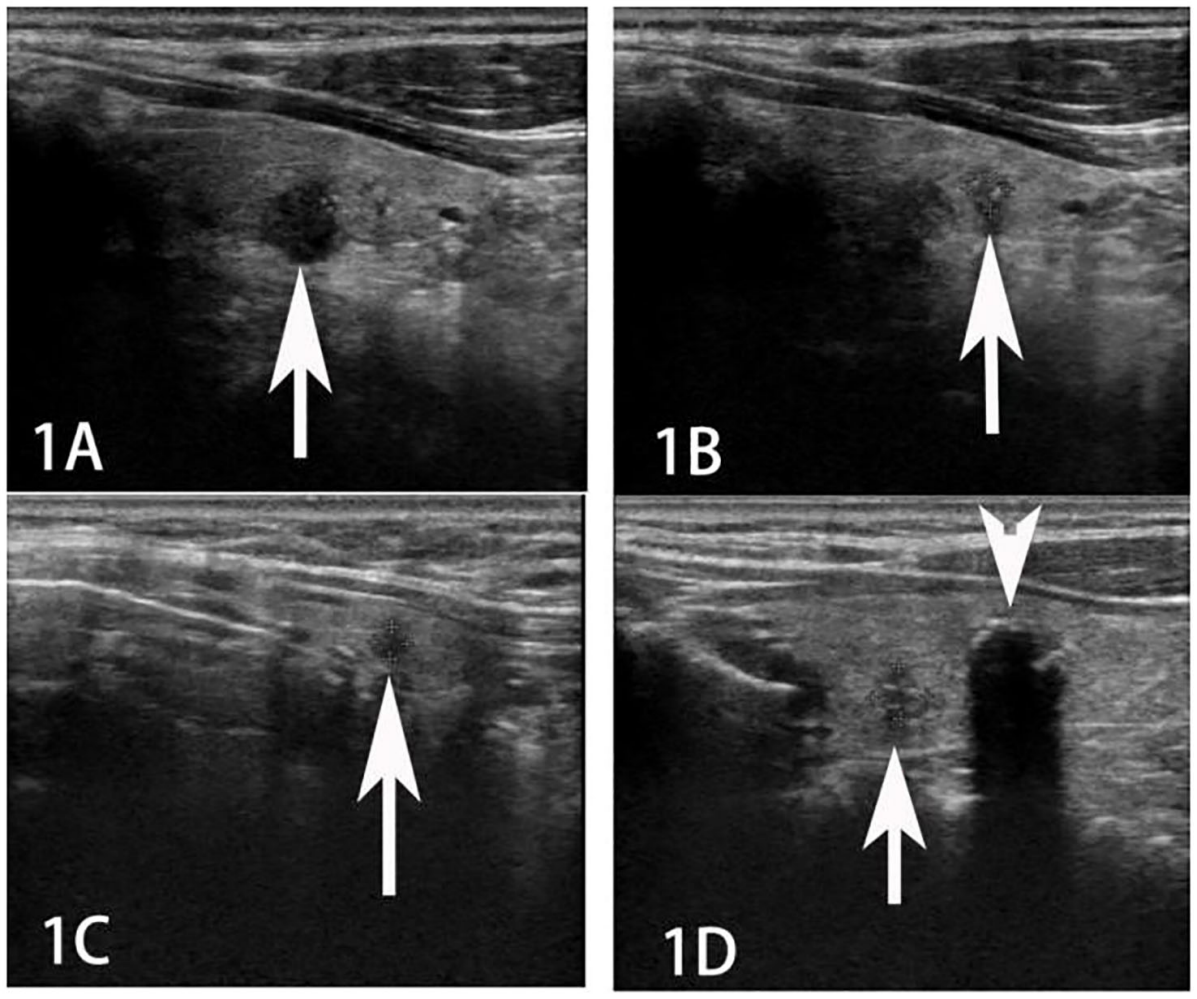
Ultrasound imaging of multiple thyroid cancers (A, B tumor in the left lobe; C tumor in the isthmus, and D tumor in the right lobe; indicated by arrows).
